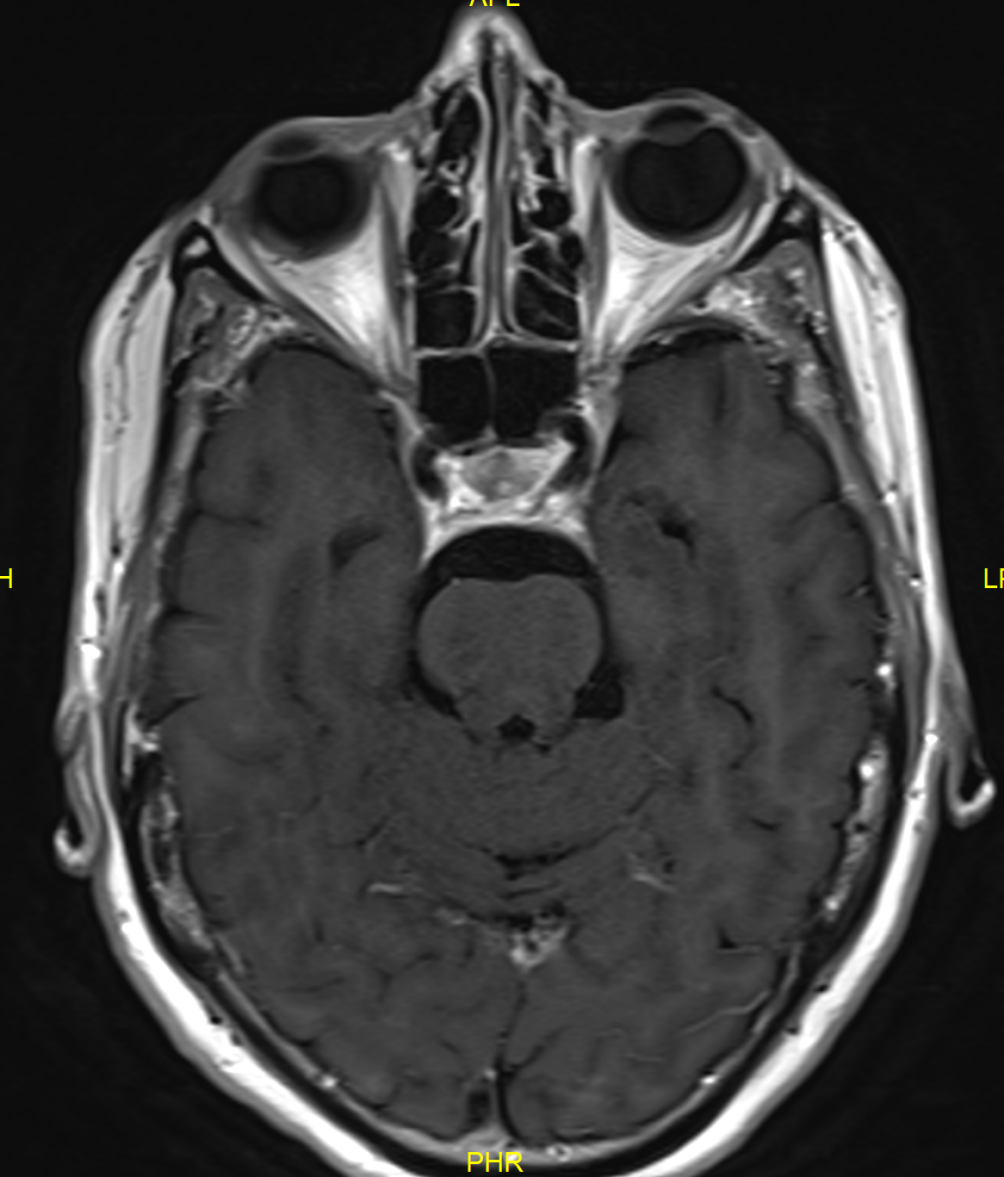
MRI (2019) at the level of the cavernous sinus
